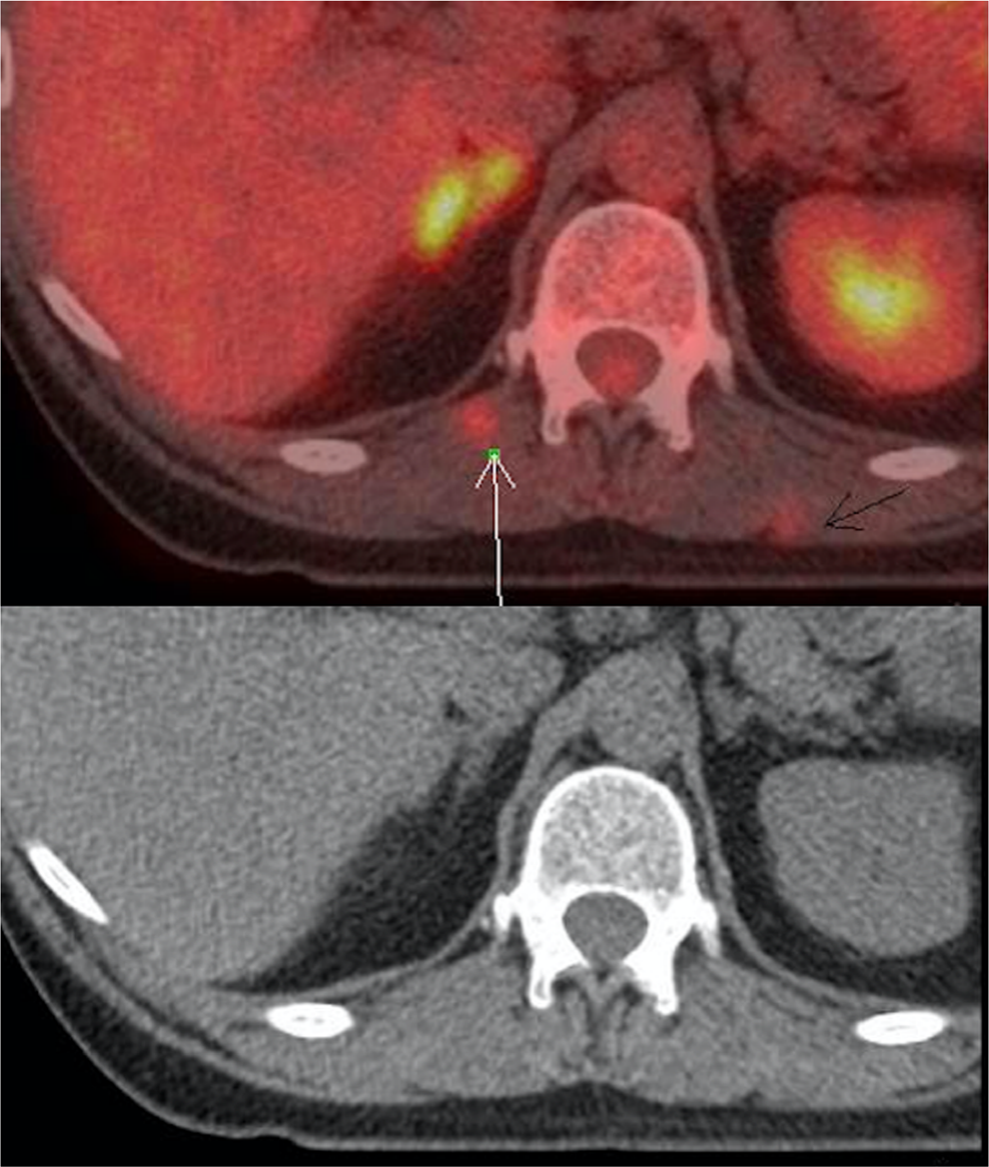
Fused PET/CT (*above*) and CT (*below*) images of soft tissue metastases (*white* and *black arrows*) in a patient initially considered suitable for curative treatment. However, the management became palliative once the metastases were detected
